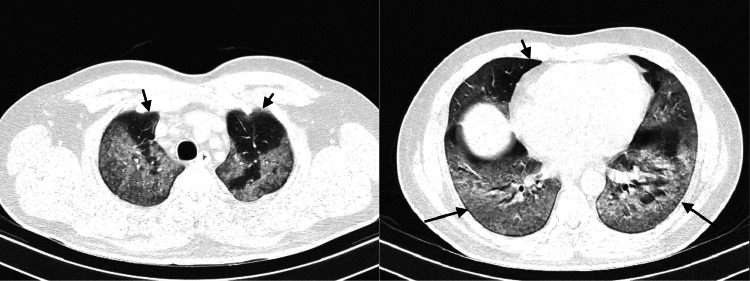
Axial CT sections with lung and soft tissue windows. The arrows show the relative sparing of anterior parts of the bilateral upper lobes and the right middle lobe.
